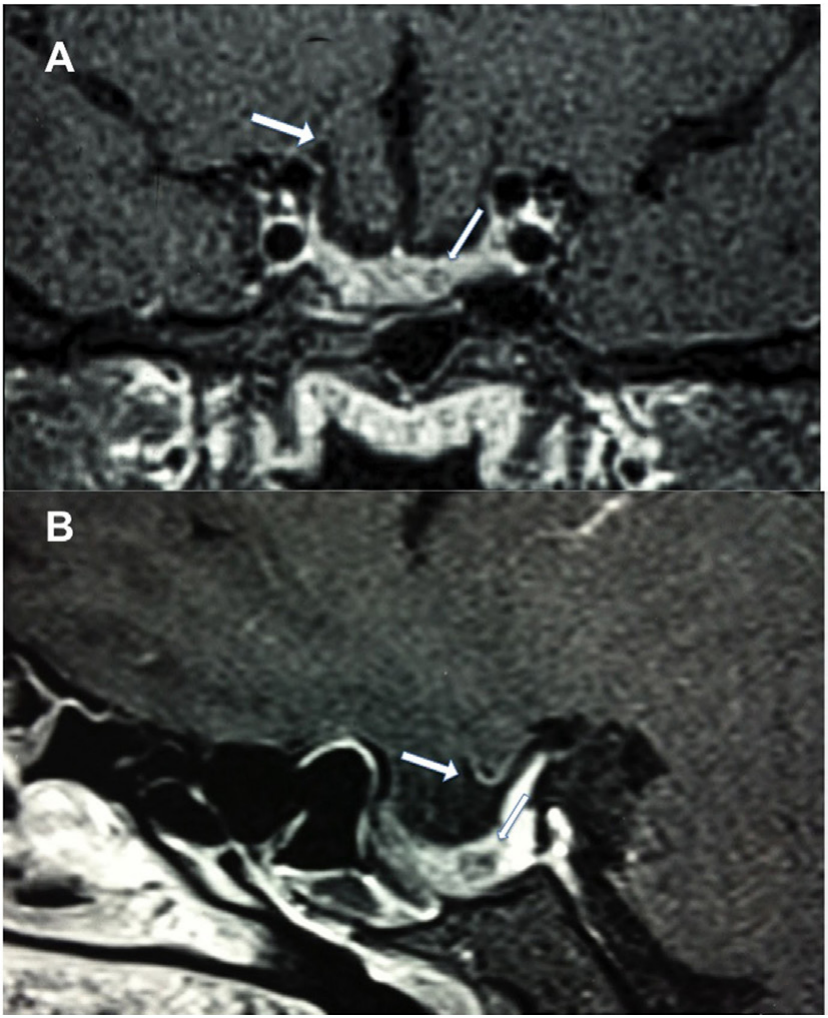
Postcontrast T1-weighted magnetic resonance imaging. (A) Coronal view showing frontal lobe herniation (thick arrow) and a 5 mm hypointense lesion (thin arrow), and (B) sagittal view showing chiasm herniation and a 5 mm hypointense lesion.
